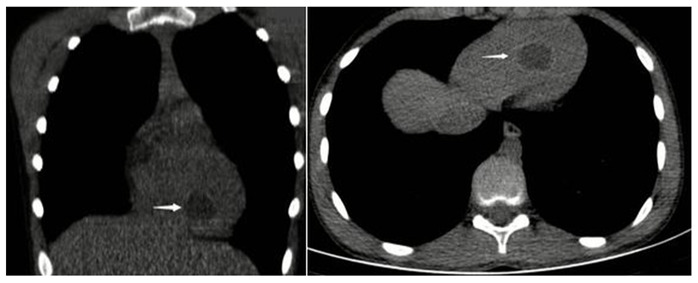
Coronal and axial computed tomography. The cystic lesion is adjacent to the interventricular septum in the heart’s left ventricle (arrow).
